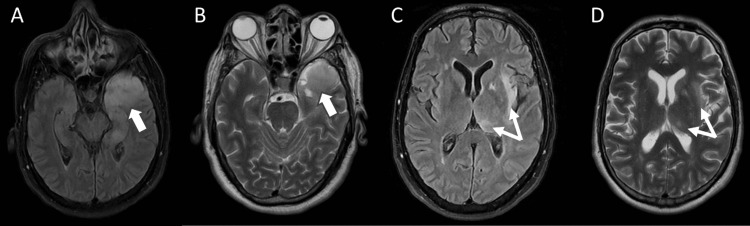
Magnetic resonance imaging (MRI) of the brain revealed extensive cortical and subcortical T2 and fluid-attenuated inversion recovery (FLAIR) hyperintensities in the left temporal lobe (A and B, thick arrows) and the left insular region, along with a focal hyperintense area in the left thalamus (C and D, thin arrows).
